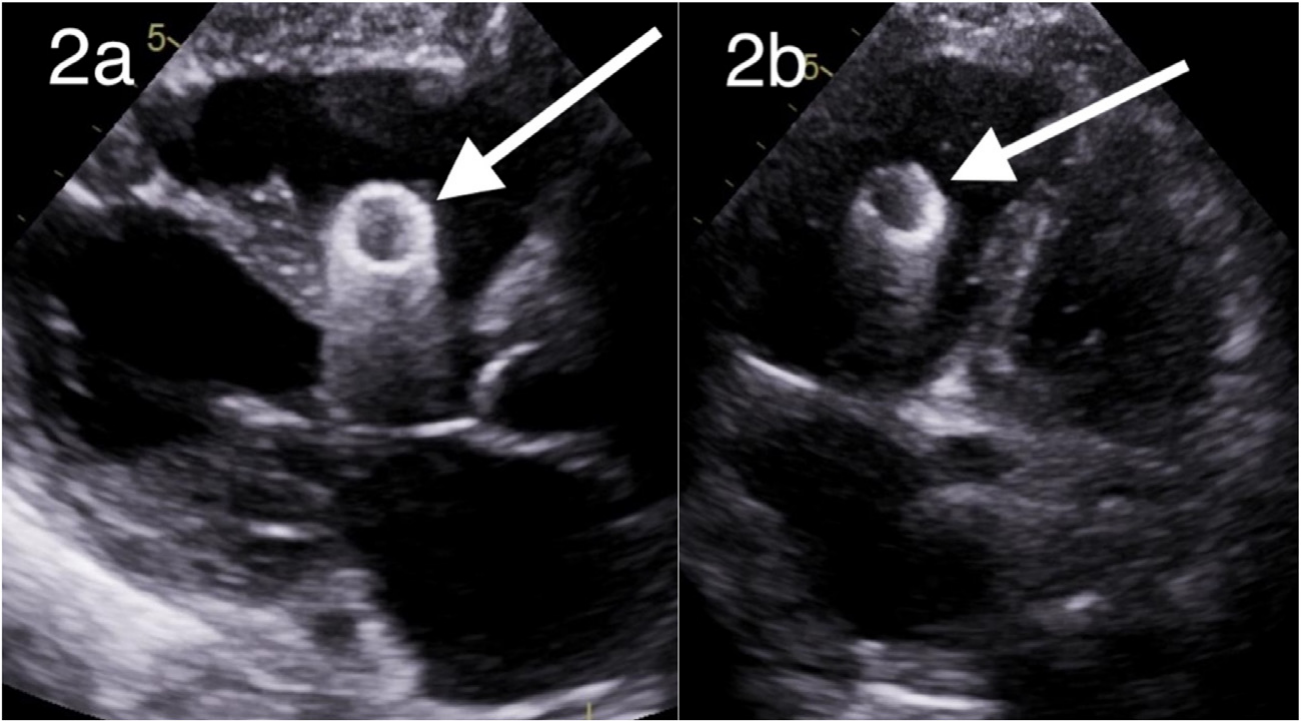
Parasternal long axis view transthoracic echocardiogram demonstrating migrated venous stent in the right ventricle (A, white arrow). Four-chamber view transthoracic echocardiogram demonstrating migrated stent in the right ventricle (B, white arrow).
